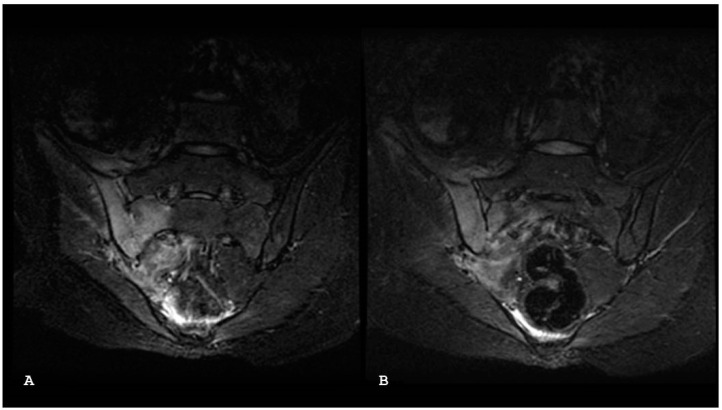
Oblique coronal short tau inversion recovery magnetic resonance imaging sequences (A,B) show diffuse bone marrow edema on both sides of the right sacroiliac joint, associated with capsulitis and enthesitis and with inflammatory fluid in the para-rectal adipose space. The spread of inflammation involving the peri-articular soft tissues supports the diagnosis of infective sacroiliitis.
